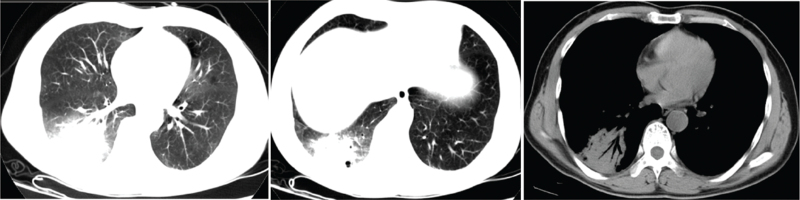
Chest computed tomography of Case 12, a 45-year-old male, showing patchy increased density shadows and consolidation shadows in the right lower lung, with air bronchograms.
